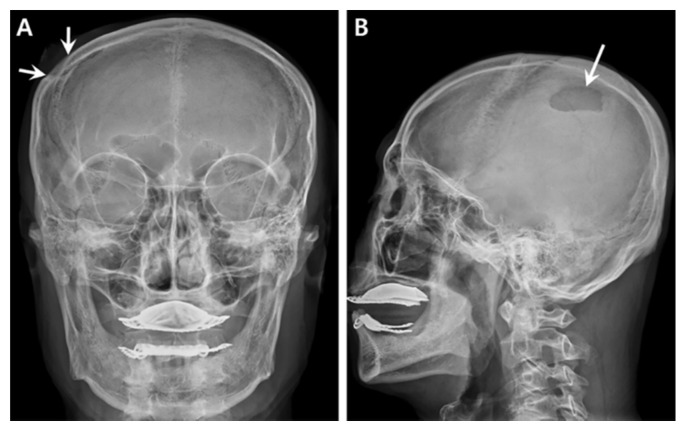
Frontal (A) and lateral (B) skull radiographs showing a well-circumscribed, oval, osteolytic lesion in the right parietal bone (arrows) with incomplete marginal sclerosis and overlying scalp swelling.
